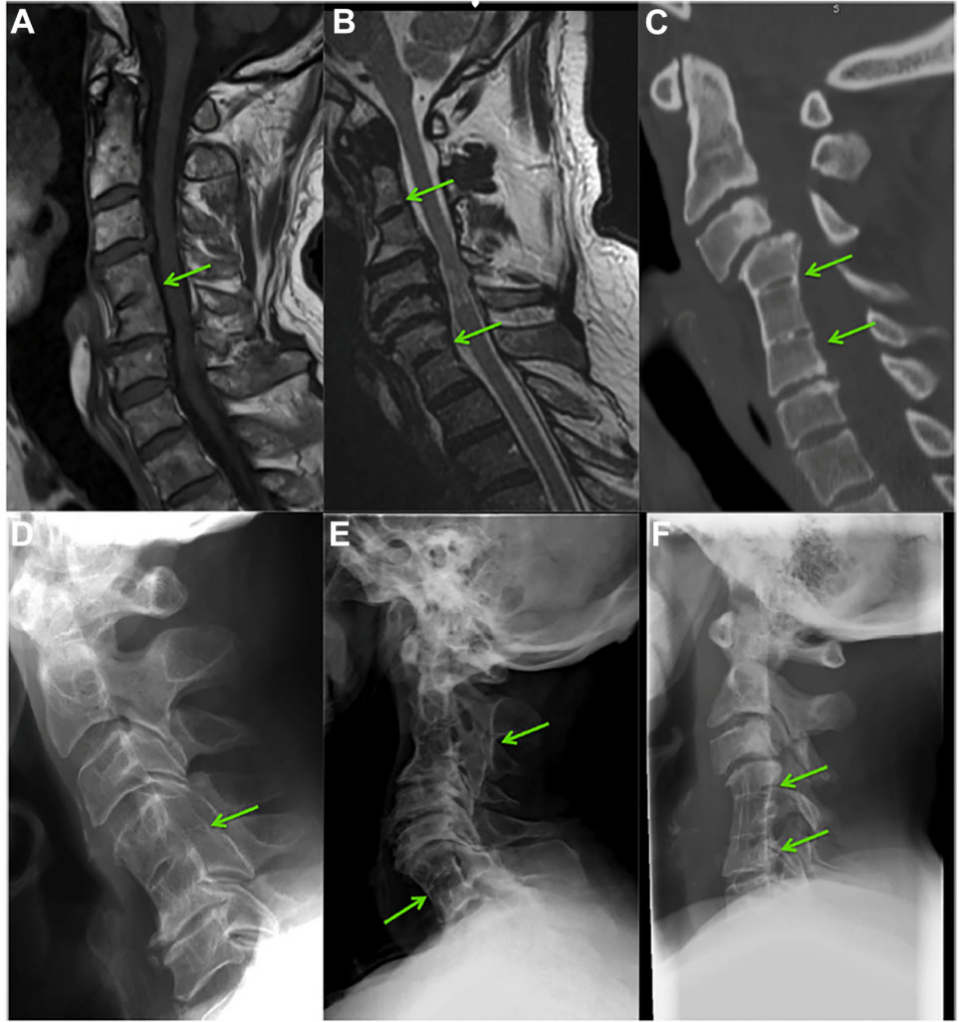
Klippel-Feil syndrome and degenerative cervical myelopathy. A and D, A single fusion of C4-5 seen on T1 MRI and lateral radiograph of the same patient. B and E, Two non-contiguous fusions between C2-3 and C6-7 on T2 MRI and lateral radiograph. C and F, Two contiguous fusions between C4-5 and C5-6 on CT and lateral radiograph. Adapted from Nouri et al.^
